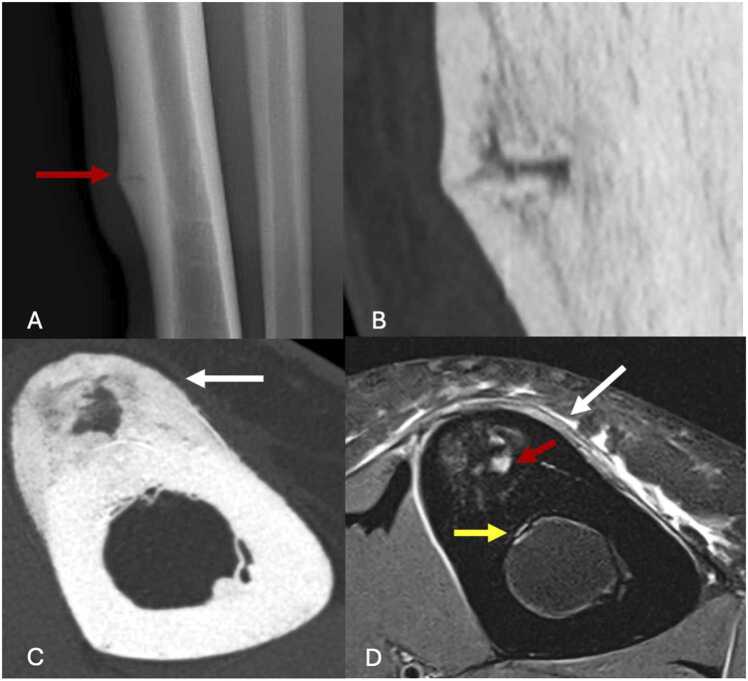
Stress fracture of the diaphysis of right tibia of a 15-year-old female adolescent playing volleyball at a high level with pain and swelling in her right leg for several months. A, Initial profile radiography, performed four months after onset of symptoms, showing cortical thickening of the distal-third of tibial diaphysis with discrete fracture line (red arrow). B, CT image in the sagittal plane highlighting the fracture line. C, CT image in the axial plane, performed with a Ultra-High Resolution CT scan, showing intense periosteal reaction and cortical thickening (white arrow). D, 2-month follow-up proton density-weighted fat-suppressed MR image in the axial plane showing periosteal (white arrow) and osteomedullar edema (yellow arrow) with fracture line (red arrow) (grade 4b of Fredericson’s classification).
